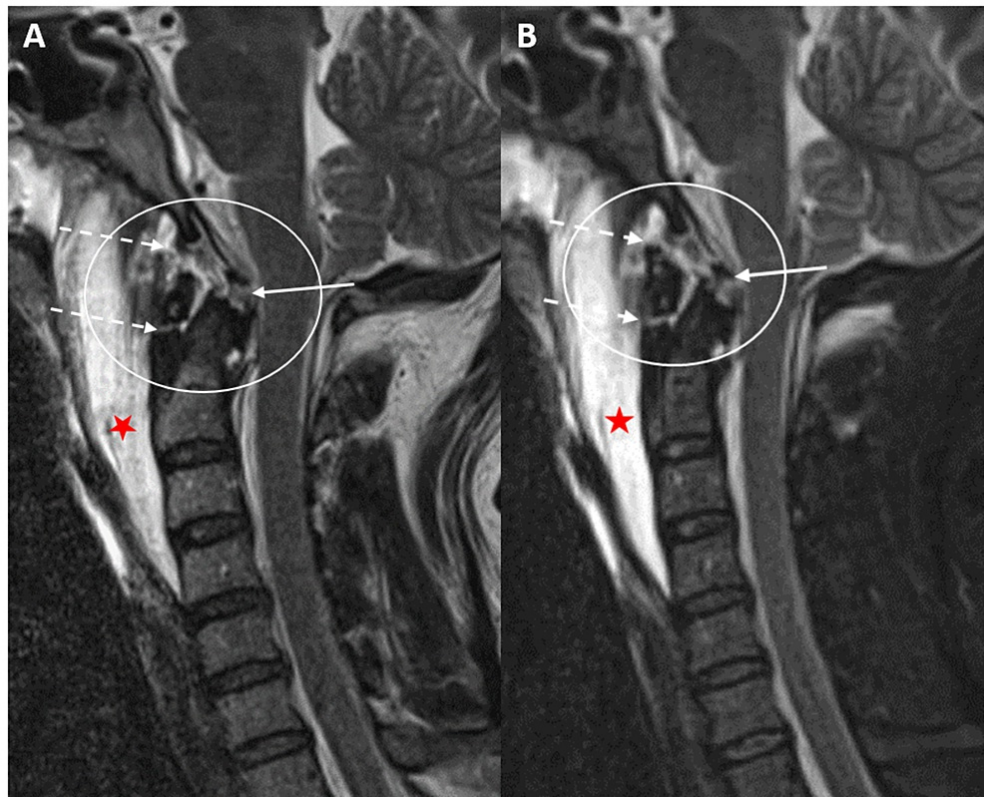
A and B: Sagittal T2 and STIR magnetic resonance imaging sequences of the craniocervical junction and cervical spine to demonstrate a subclival tectorial membrane tear (white arrow) in a 49-year-old male status post automobile vs. pedestrian incident. Additional tears of the anterior atlantooccipital membrane and anterior longitudinal ligament at C2 (dashed arrows) with a large prevertebral effusion (red star). STIR = short tau inversion recovery
